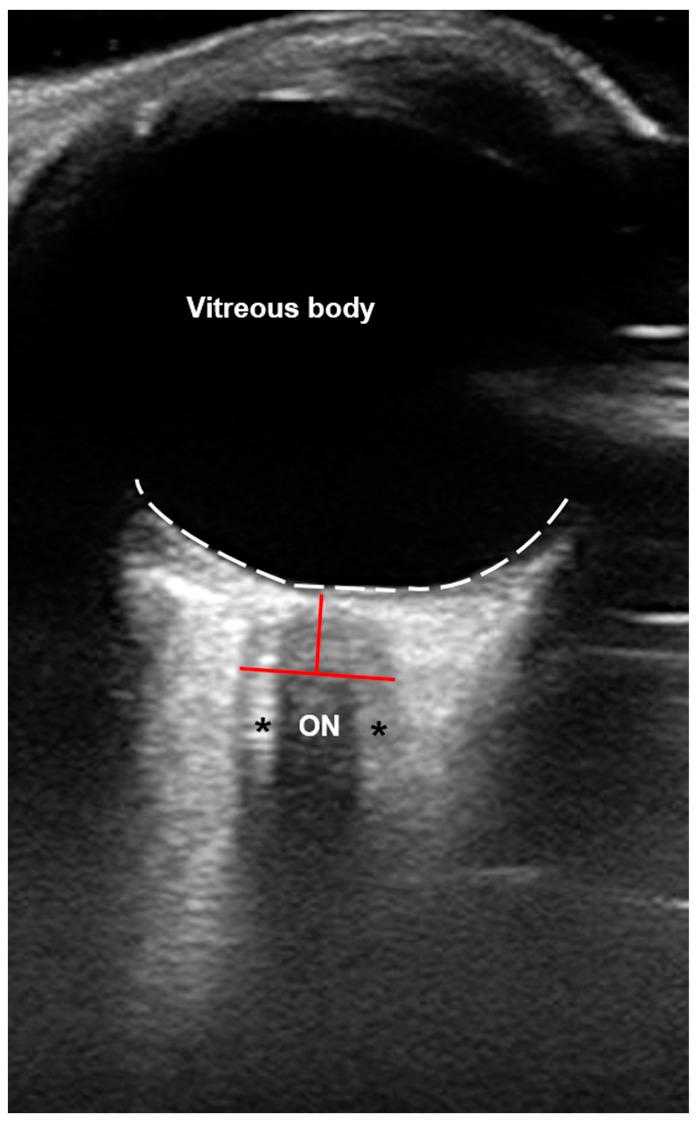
Axial B-scan ultrasound (US) of the orbit. The optic nerve (ON) appears hypoechogenic and is surrounded by the ON sheath (ONS; black asterisks) that encases the optic subarachnoid space (SAS), which is filled with cerebrospinal fluid (CSF) and subdivided by multiple trabeculae [22], presenting with a hyperechogenic appearance. The white dotted line marks the retina. Red lines mark the exact region to measure the ONS diameter (ONSD) at about 3 mm posterior to the ON head.
